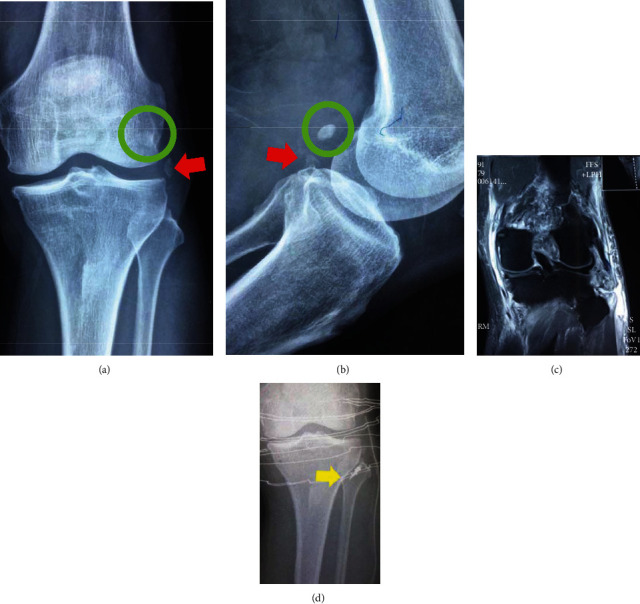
A type 1 fibular column injury case: (a, b) the preoperative X-ray; (c) the MRI shows avulsion of lateral collateral ligament; (d) the avulsion fracture of fibular apex was fixed by suture anchor. Green cycle: sesamoid; red arrow: avulsion fracture of fibular apex; yellow arrow: metal anchor.
